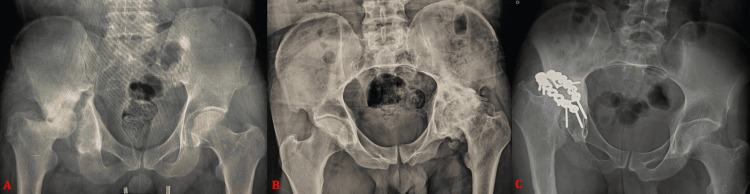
(A) Anteroposterior pelvic radiograph showing sequelae of a transverse fracture of the right acetabulum treated conservatively, with progression to fracture non-union and avascular necrosis of the femoral head in a 31-year-old male patient. (B) Anteroposterior pelvic radiograph demonstrating left post-traumatic hip osteoarthritis associated with heterotopic ossification following a posterior wall fracture-dislocation treated conservatively in a 58-year-old male patient. (C) Anteroposterior pelvic radiograph showing a right acetabular fracture treated by posterior open reduction and internal fixation using two plate-and-screw constructs, with progression at 46 months to post-traumatic hip osteoarthritis associated with femoral head avascular necrosis in a 47-year-old male patient.
